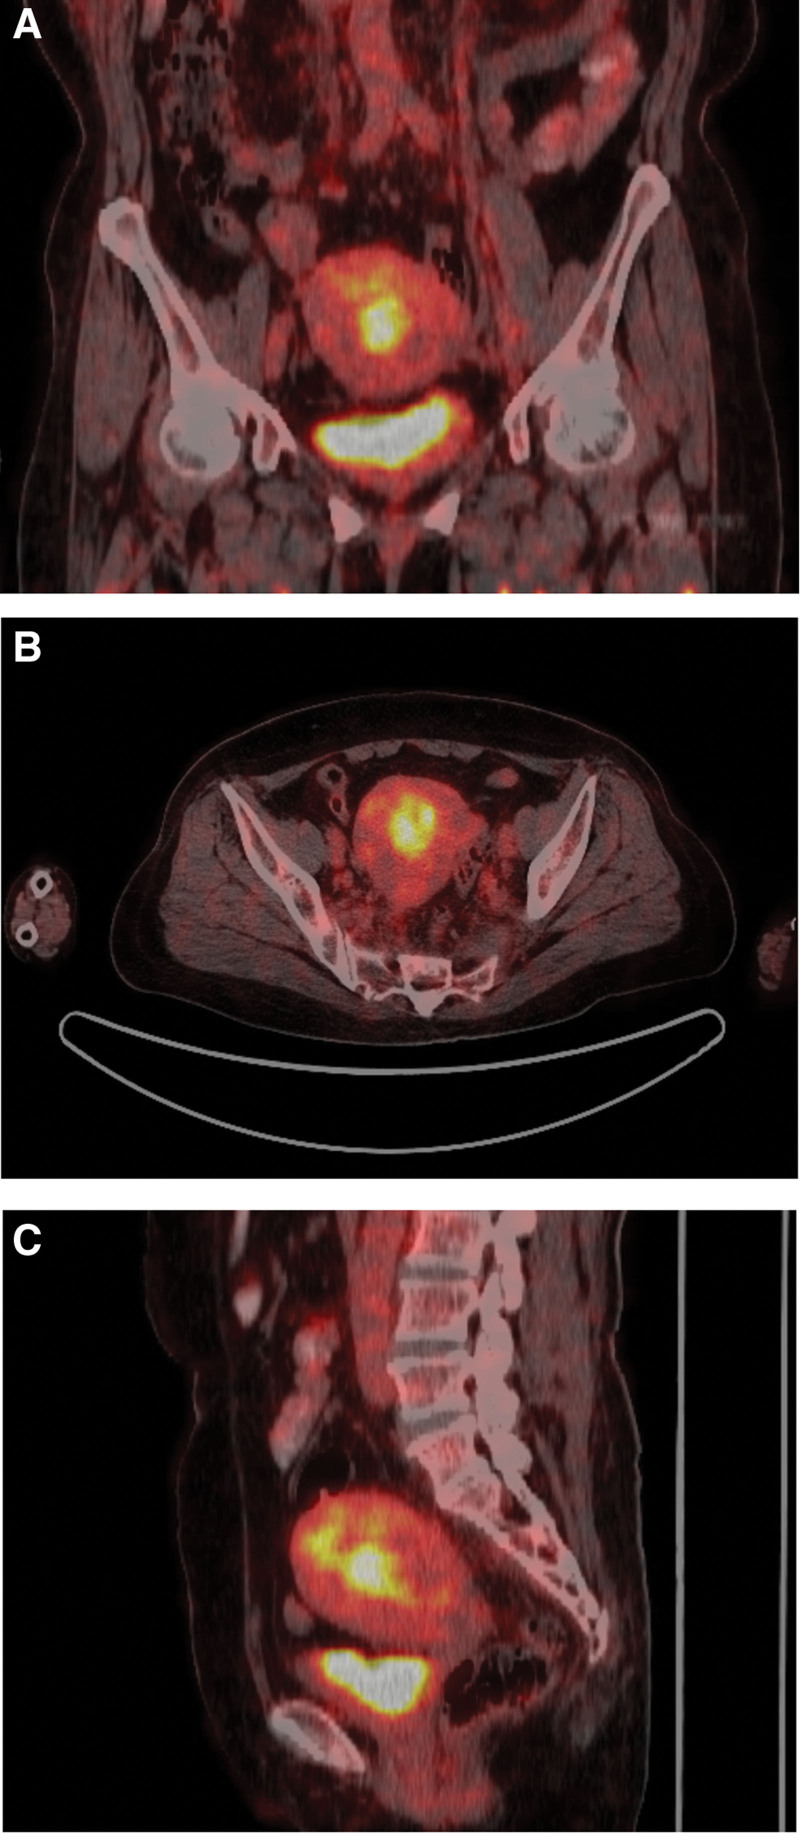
Abnormal high metabolic signal of the uterus by PET-CT examination. (A: PET/CT pelvis – axial view; B: PET/CT pelvis – coronal view; C: PET/CT abdomen & pelvis – sagittal view).
